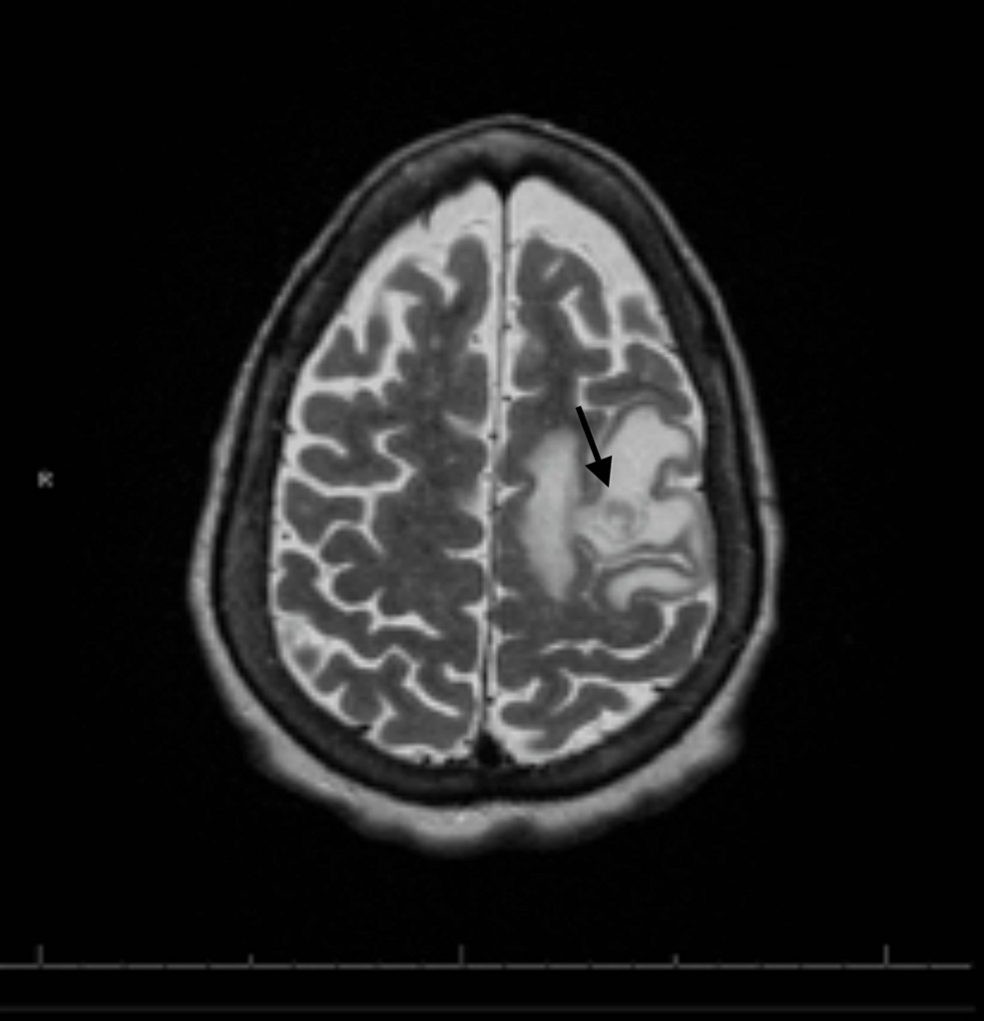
Magnetic resonance imaging of the brain on T2/FLAIR (fluid attenuated inversion recovery) sequence demonstrating central necrotic core characteristically observed in glioblastoma multiforme.
